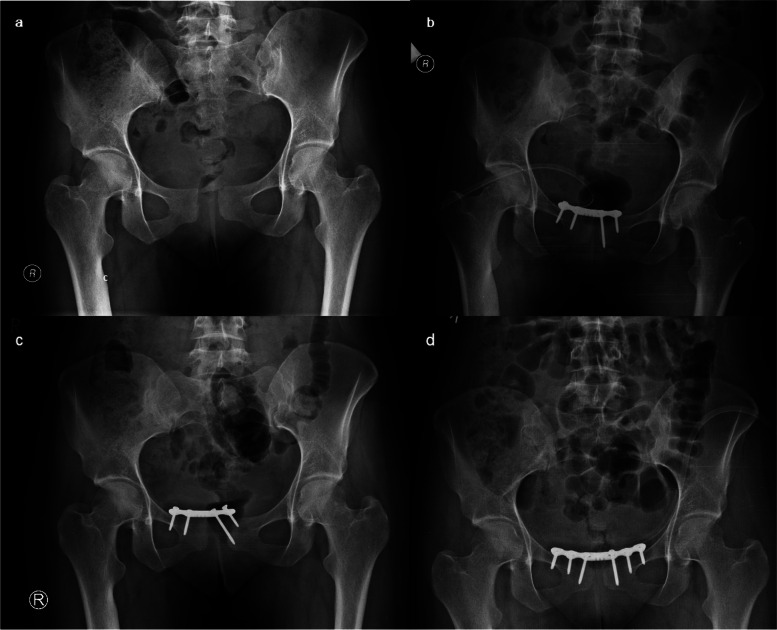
Case 3. Four months postpartum, a 30-year-old female was admitted to the department with pain in the pubic region. a Initial plain radiograph showing rupture of pubic symphysis with diastasis of 22 mm in width and 4 mm vertical. b The immediate postoperative film after open reduction and internal fixation of the pubic symphysis with a 4-hole plate. c Three-month follow-up radiograph showing plate failure. d The immediate postoperative film after revision surgery
